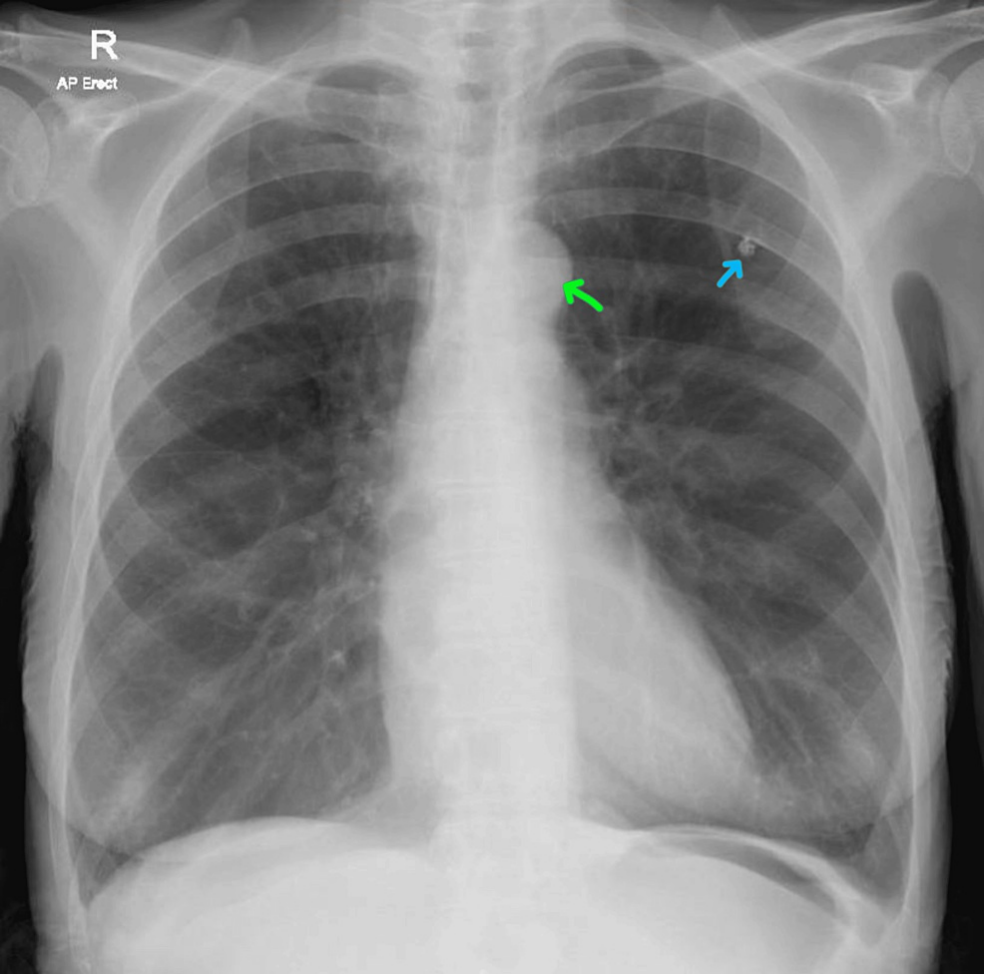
An erect chest X-ray shows lung hyperinflation with a flattened diaphragm consistent with chronic obstructive pulmonary disease, a calcified granuloma at the left upper zone (blue arrow), and aortic knob enlargement (green arrow) consistent with her long history of hypertension. AP, anteroposterior
